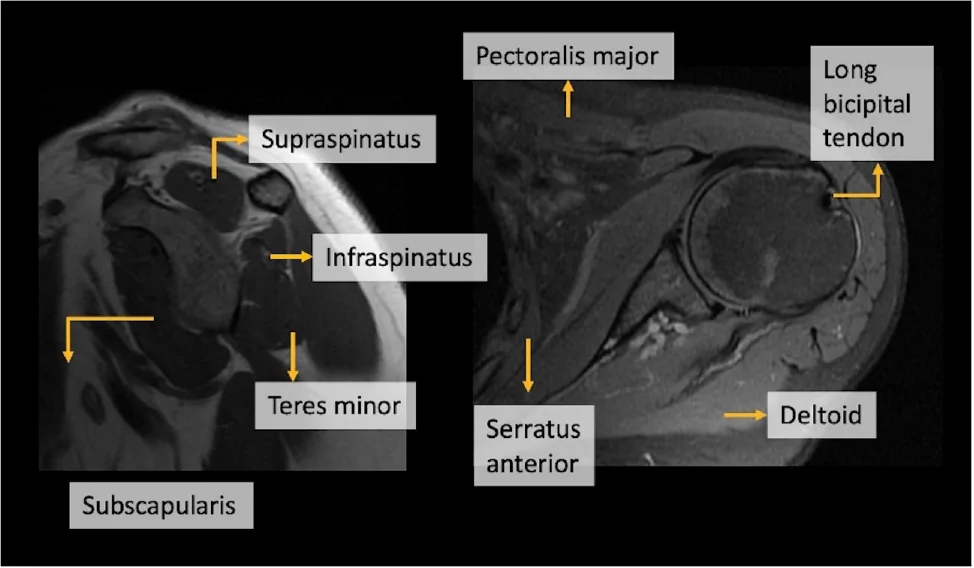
CT scans of the muscles surrounding the shoulder joint. The rotator cuff
